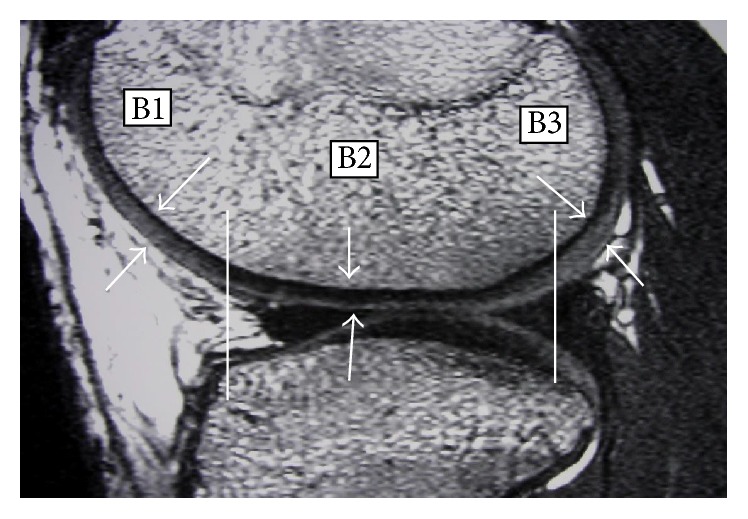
Mapping of MRI showing regions that are B1, B2, and B3. White arrows show cartilage thickness in these three regions.
